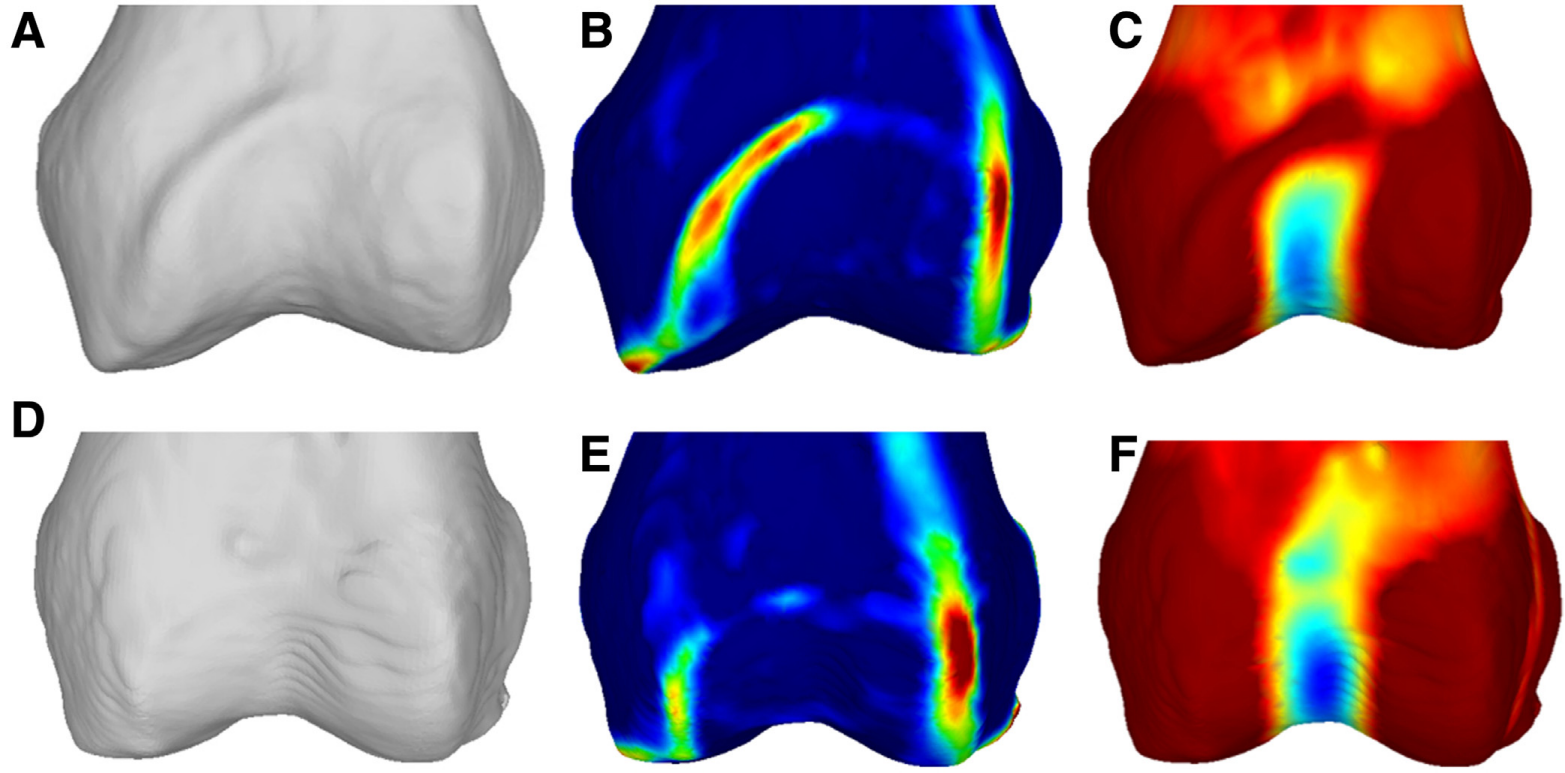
(A) Anteroposterior (AP) view of a 3-dimensional model created from a patellofemoral instability patient computed tomography scan. (B) AP view highlighting small structures with a high curvature (i.e., medial and proximal trochlear ridge). (C) AP view highlighting structures with a low curvature. (D-F) The corresponding AP views for a typical control. Typically, the proximal ridge in the patient cohort is more lateralized, and the trochlear groove terminates earlier, is lateralized, and is shallower.
